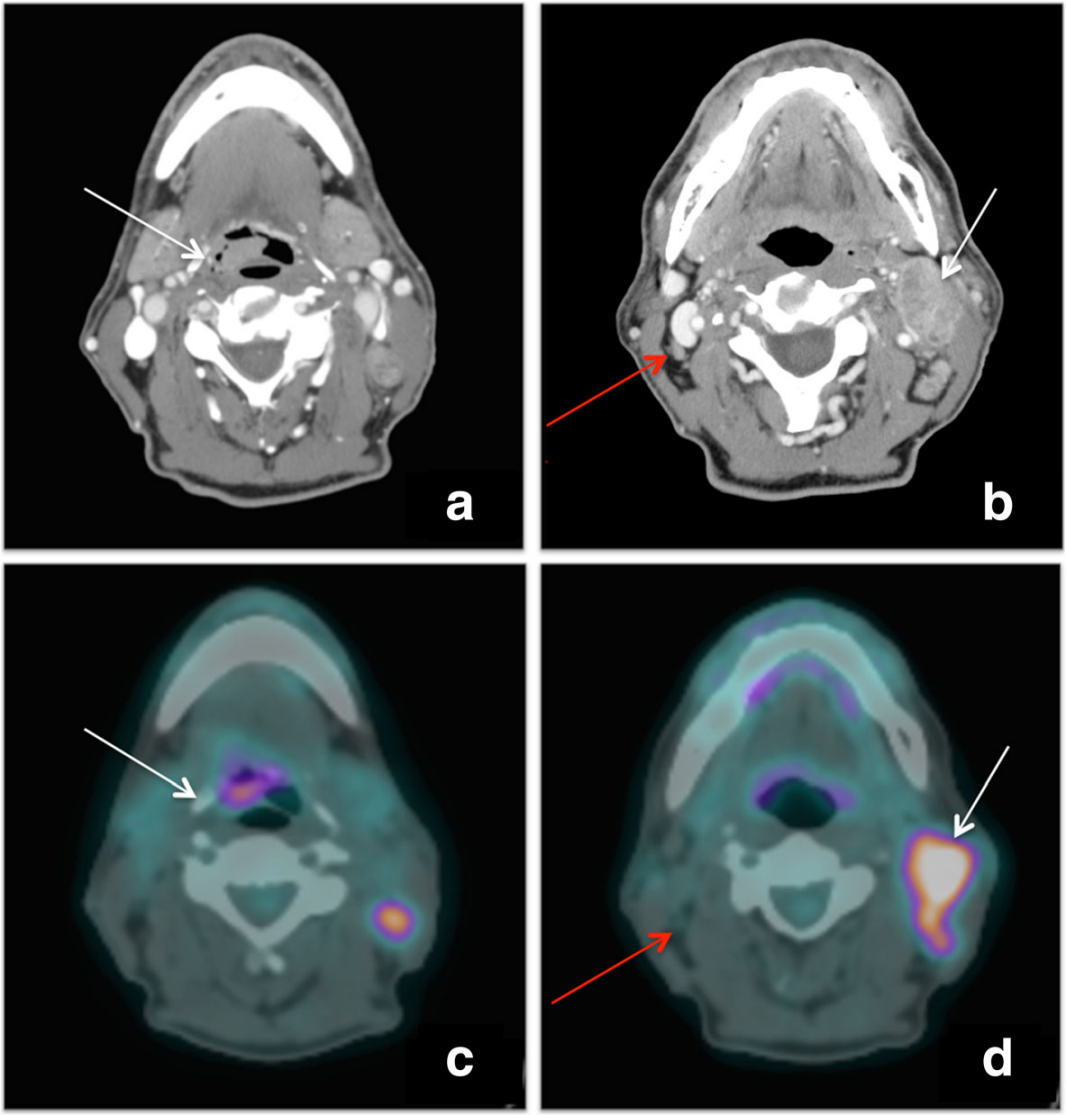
a transaxial c.e.CT: primary epiglottic lesion. b transaxial c.e.CT: lateral cervical lymph nodes. c transaxial PET/CT: hypermetabolic primary epiglottic lesion. d transaxial PET/CT: not pathological right lateral cervical lymph nodes, left hypermetabolic lateral cervical adenopathy
